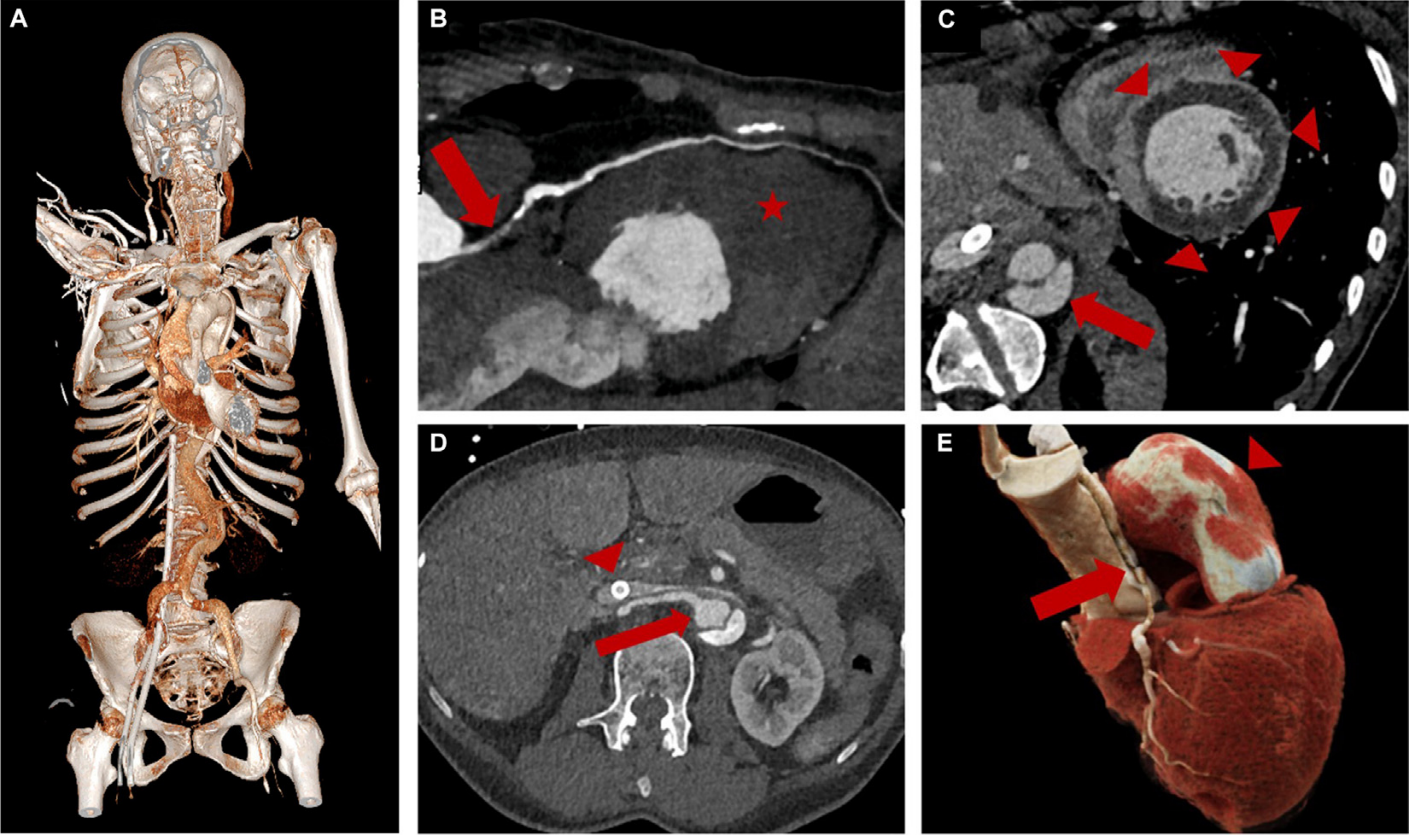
(A) Computed tomography images in three-dimensional rendered format showing the aortic dissection extending from the aortic root to the iliac bifurcation, with the venous cannula positioned in the inferior vena cava and the arterial cannula in the right iliac artery, located within the true lumen. (B) Dissection (arrow) of the left anterior descending artery along its entire length, resulting in a large myocardial infarction involving the entire left ventricle (star). (C) Short axis cardiac view showing a large myocardial infarction of the left ventricle (arrowheads) and dissected abdominal aorta (arrow). (D) Venous cannula located in the inferior vena cava (arrowhead), with the right renal artery arising from the true lumen (arrow) of the dissected abdominal aorta. (E) Left-sided view of a three-dimensional reconstruction (Siemens Healthineers Syngo.via) of the heart, ascending aorta and left anterior descending artery (LAD), showing enlarged diameter of the ascending aorta due to ATAAD (arrowhead) and stenotic LAD (arrow), caused by the extension of the ascending aorta dissection. 3D, three-dimensional; ATAAD, acute type A aortic dissection.
